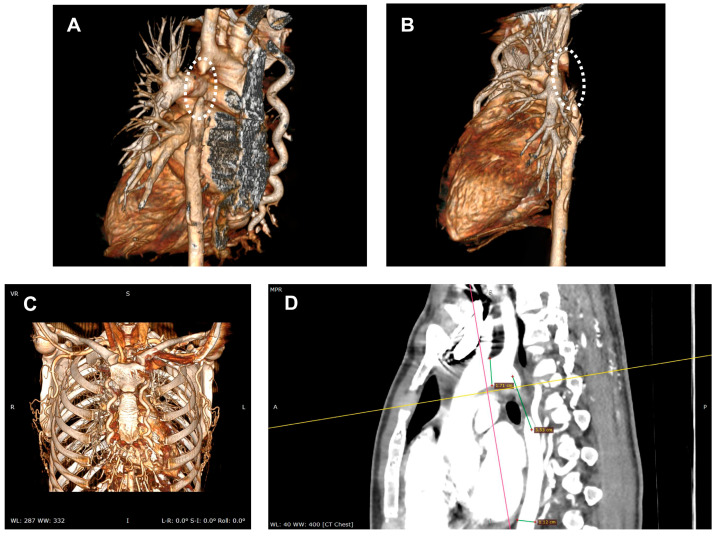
(A,B). Computed tomography angiography with three-dimensional reconstruction identified an atretic segment in the descending thoracic aorta (dashed circle). (C). Computed tomography angiography with three-dimensional reconstruction demonstrating a well-developed collateral circulation between the proximal and distal portions of the atretic aortic segment; (D). Sagittal view showing a proximal aortic diameter of 1.71 cm, a descending aortic diameter of 1.12 cm, an atretic segment measuring approximately 2.5 cm in length, and a stent landing zone of 3.53 cm.
